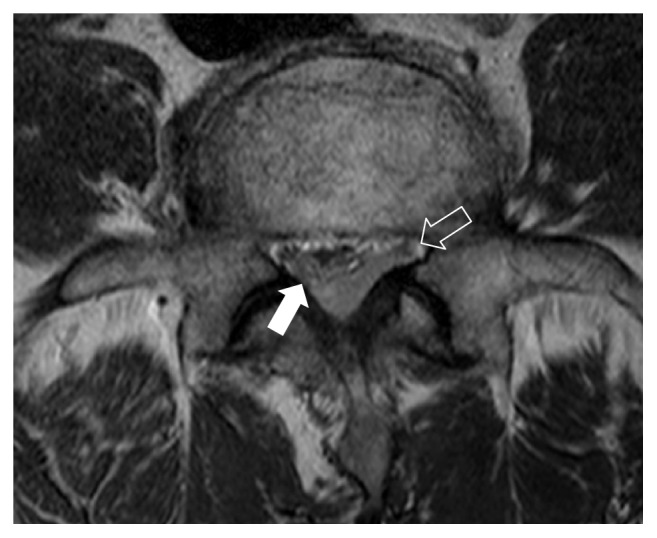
Axial T2-weighted image in upper body level of L5 reveals compression of thecal sac (solid arrow) and left S1 nerve root (open arrow).
